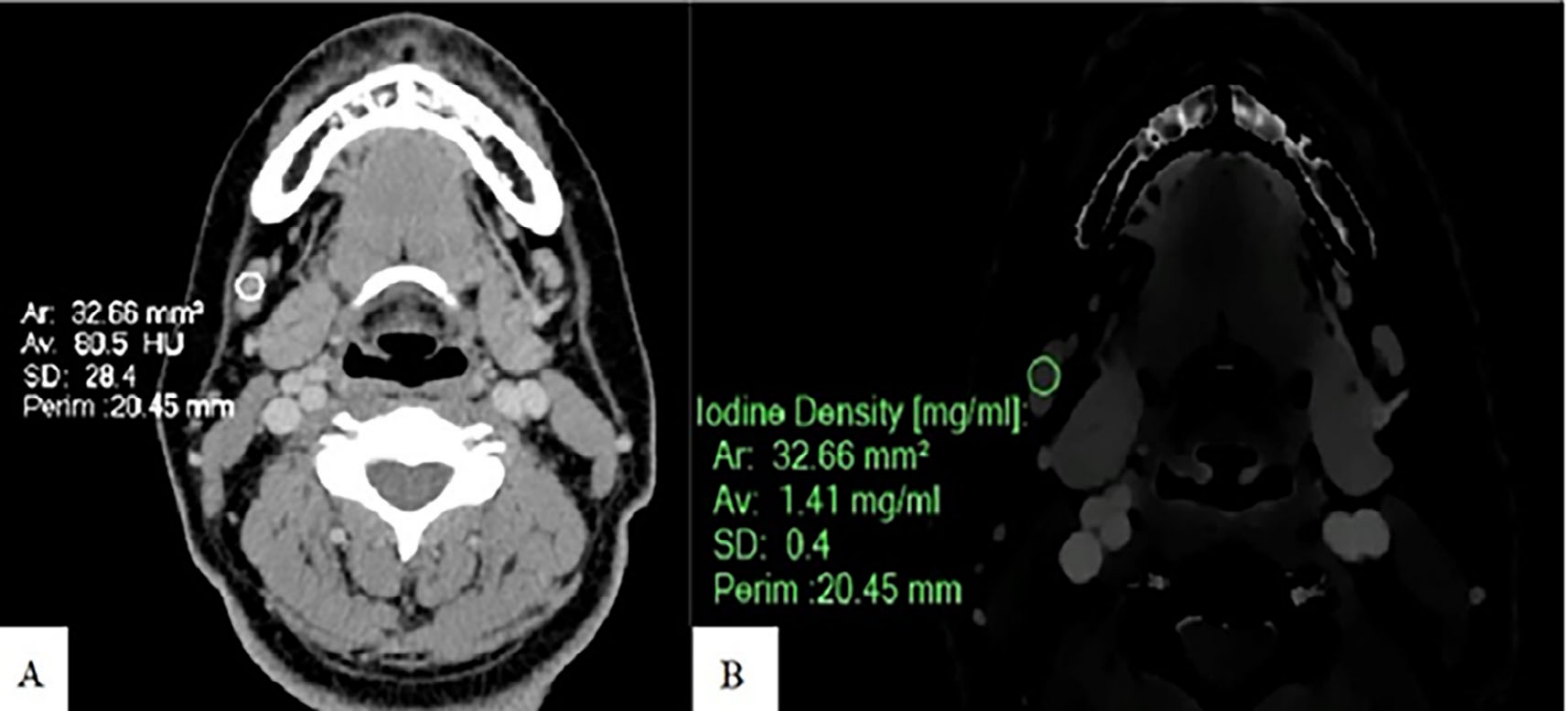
Measurement of ID and CEAV in normal node. An Axial CT image of a patient with normal lymph nodes shows CEAV measurement (80.5 HU) of a right cervical level IB node (A). An ID measurement (1.41 mg/ml) of this lymph node at the same CT level (B).
